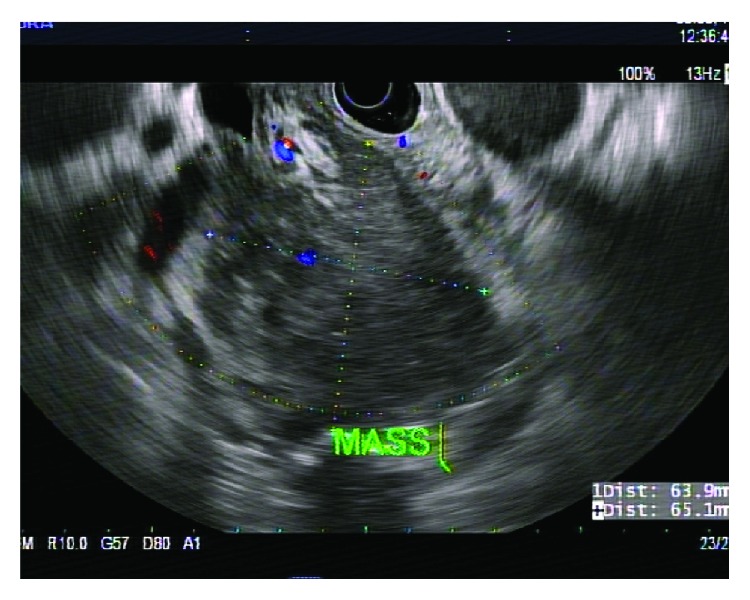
Endosonographic image demonstrating a well-defined round, hypoechoic mass, measuring 65 mm × 64 mm in maximal cross-sectional diameter.
